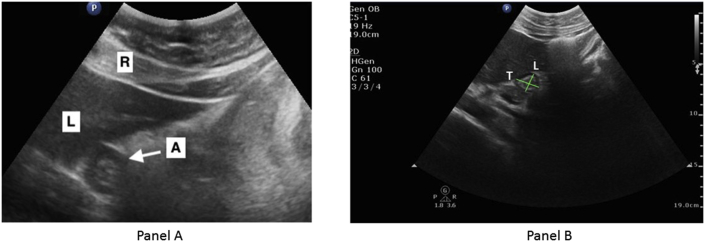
Transverse ultrasonographic view of gastric antrum (A arrow) in relation to the liver (L) and rectus abdominis muscle (R) (Panel A). Cross-sectional antral diameters.L, longitudinal cranio-caudal axis.T, transversal axis (Panel B).
